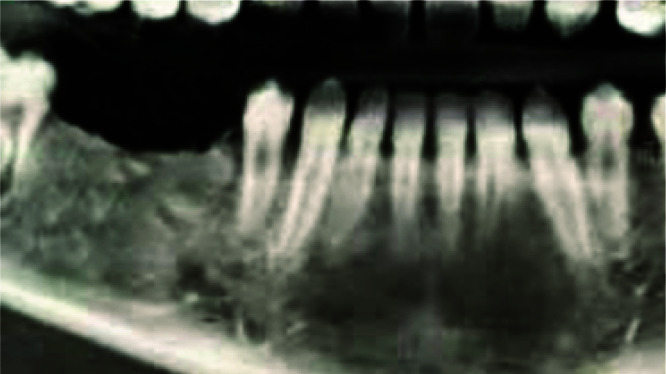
Dental panoramic radiograph of the patient at follow up revealed obviously bone repair of alveolus
